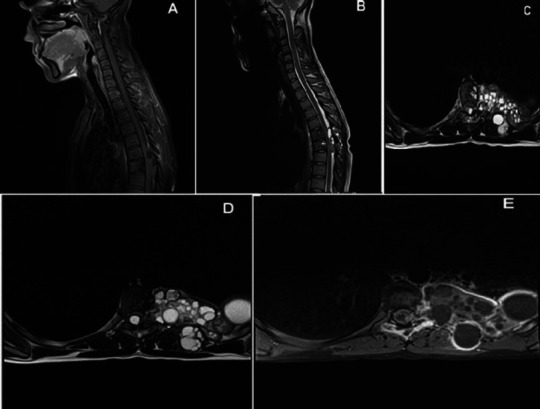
spinal magnetic resonance imaging: A) sagittal T1-weighted image, the lesions appear hypointense on T1-weighted images; B) sagittal T2-weighted image, hyperintense on T2-weighted images; C, D) axial T2-weighted images, hyperintense on T2-weighted images; E) post-contrast T1-weighted axial image: multilobulated lesion with both solid and multivesicular components, centered on the left costo-vertebral groove at the D6-D7 level, with peripheral contrast enhancement
